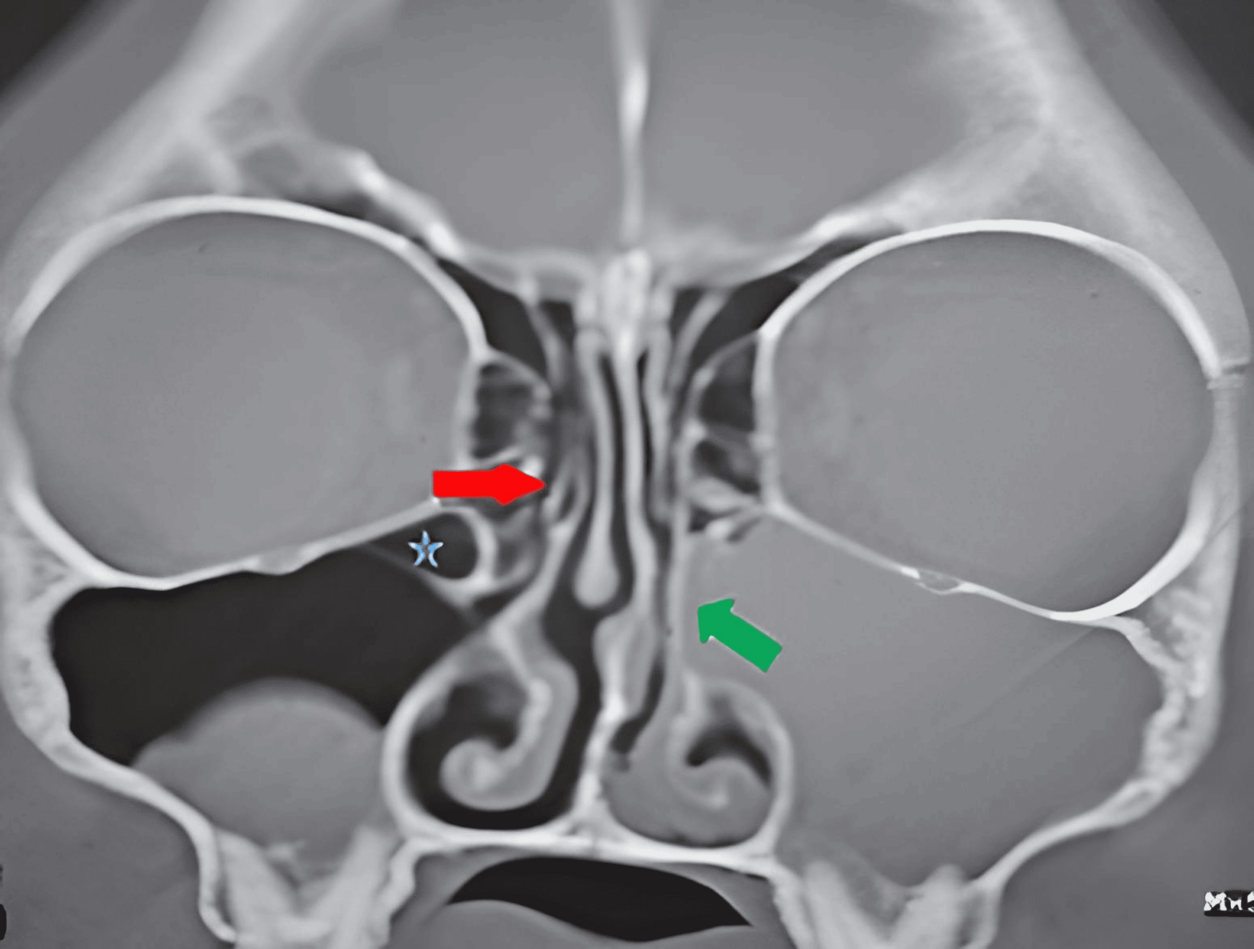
Non-injected coronal section CT scan: left antrochoanal polyp with pneumatized right unciform process (red arrow), right Haller cell (blue star) and left verticalized unciform process (green arrow) Here is a representative image for our cohort showing a non-injected coronal section CT scan: left antrochoanal polyp with pneumatized right unciform process (red arrow), right Haller cell (blue star) and left verticalized unciform process (green arrow).
